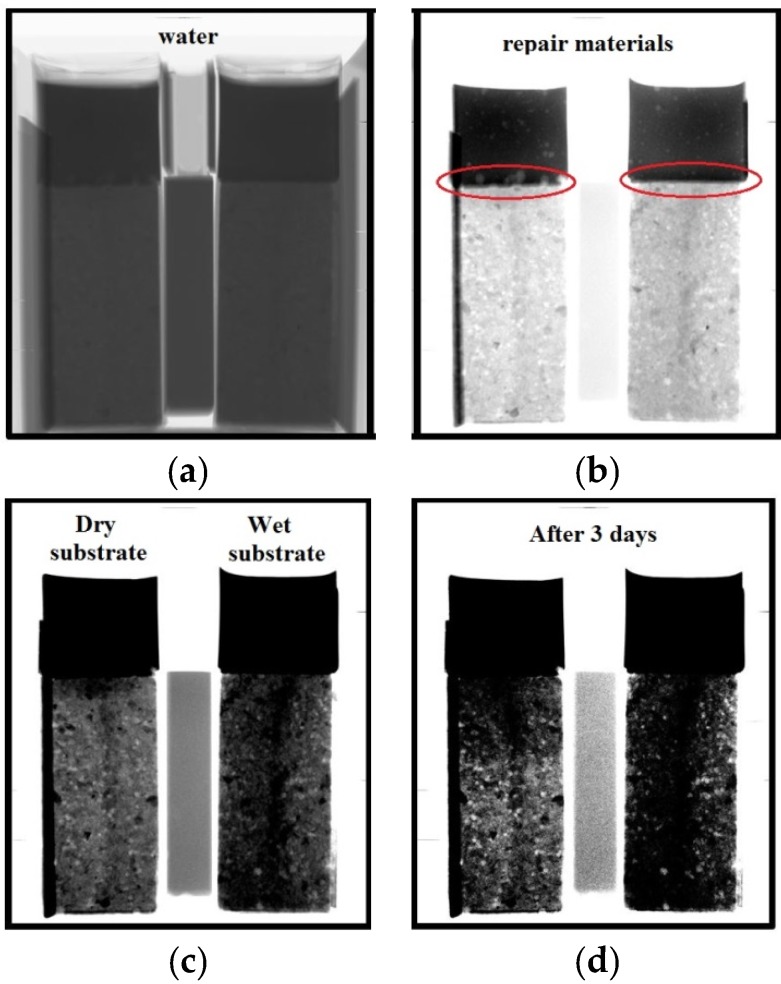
X-ray measurements on repair systems (w/c = 0.3, DS, S3 and w/c = 0.3, WS, S3): (a) Reference image; (b) Chosen GSV range with the emphasis on the repair material; (c) Chosen GSV range with the emphasis on the mortar substrate; and (d) Image after 3 d of sealed curing.
